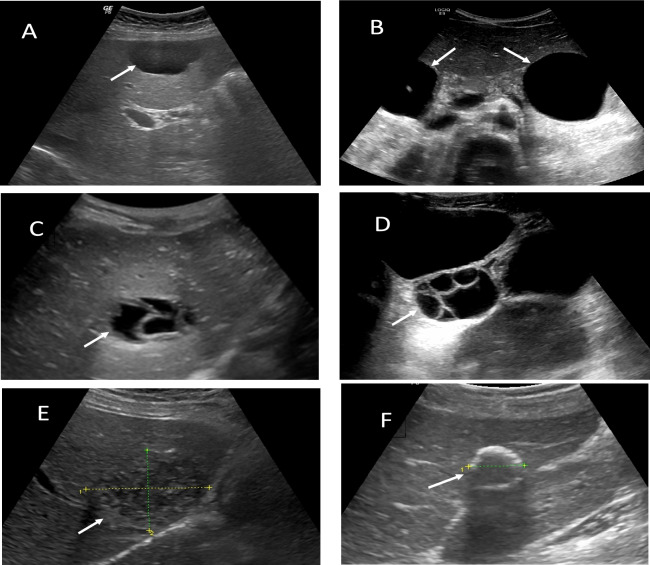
Ultrasonographic appearances of hepatic hydatid cysts according to Gharbi's classification (cysts are shown with arrows): A & B. Type 1; Pure fluid collection, C. Type 2; Fluid collection with a split wall, D. Type 3; Fluid collection with septa, honeycombed or with daughter vesicles E. Type 4; Reflecting thick walls, heterogeneous solid mass F. Type 5; Heterogeneous echo patterns, calcified hyperechoic lesion
